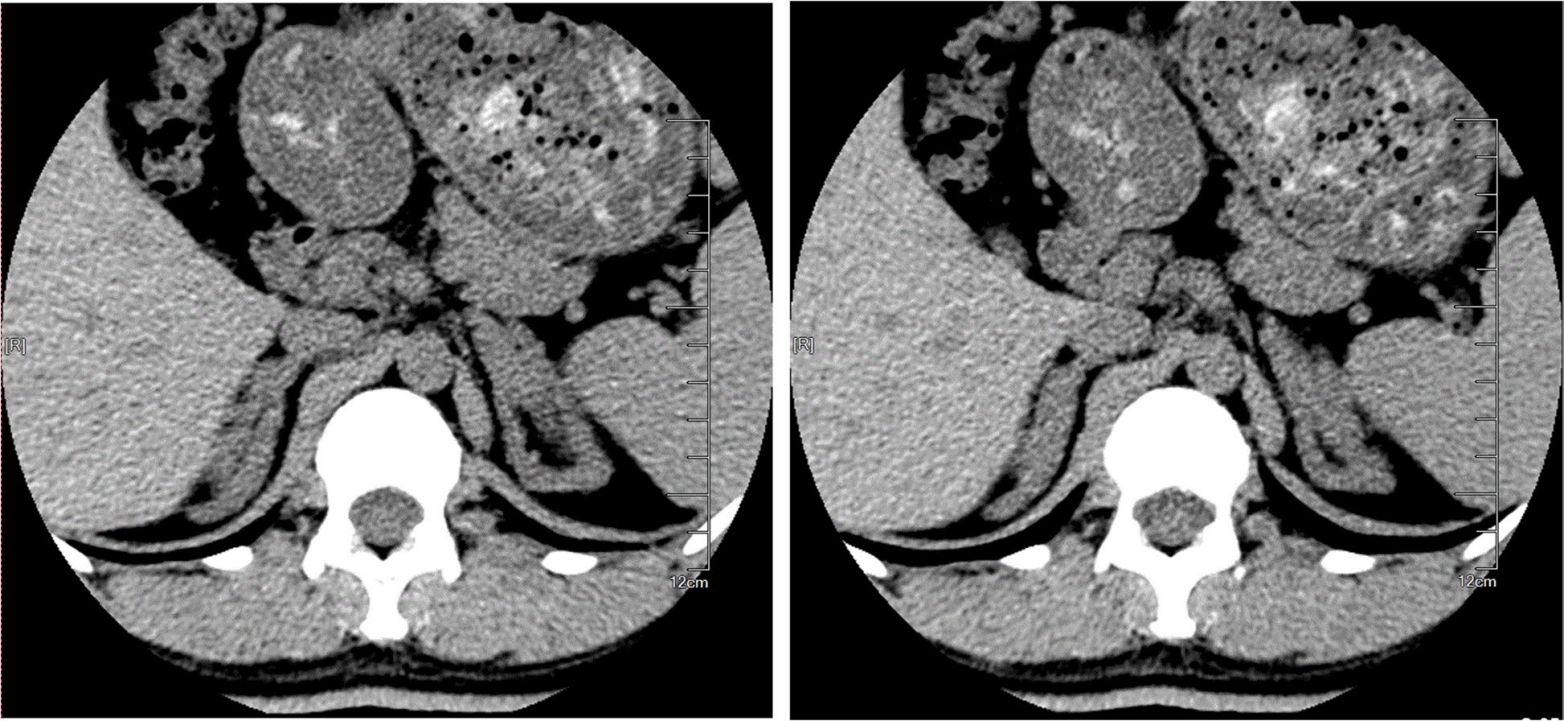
Adrenal computed tomography of the proband showing an increased bilateral adrenal volume
